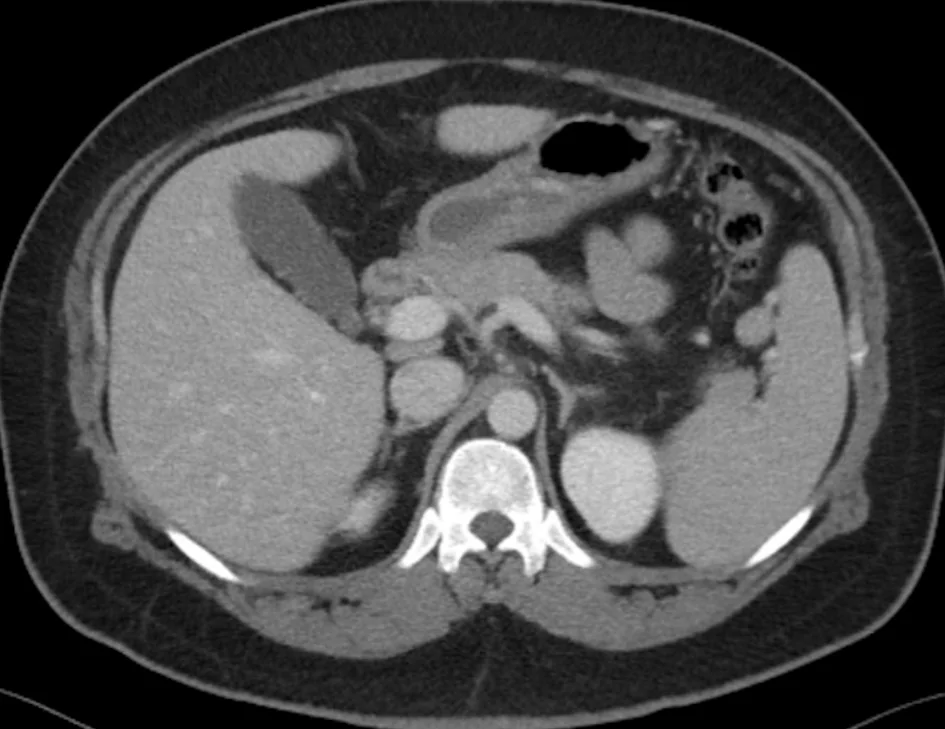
Follow-up contrast-enhanced axial CT image of the abdomen obtained 16 weeks after admission, showing complete radiological resolution with no residual hepatic lesions. CT: computed tomography.
